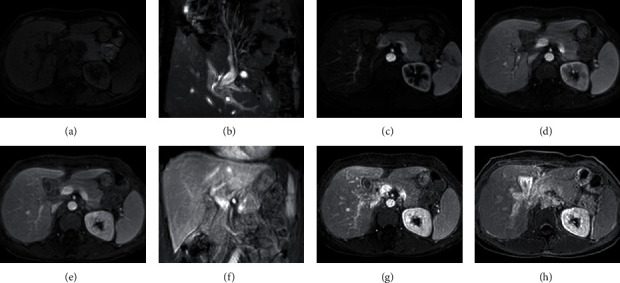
MRI LAVA dynamic enhanced scanning showed unclear hilar features. A 53-year-old female patient with hilar cholangitis had poor breath-holding coordination, and some phase images were not clearly displayed due to the presence of respiratory artifacts. Scan and T2WI coronary (a and b), no obvious abnormal changes; LAVA dynamic enhanced early periods image quality display fair, no obvious respiratory motion artifact (c–h); hepatic portal vein in the bile duct wall thickening of mild change, late to enhance image has the obvious respiratory motion artifact, hepatic portal vein display is not clear, cannot be used for diagnosis.
